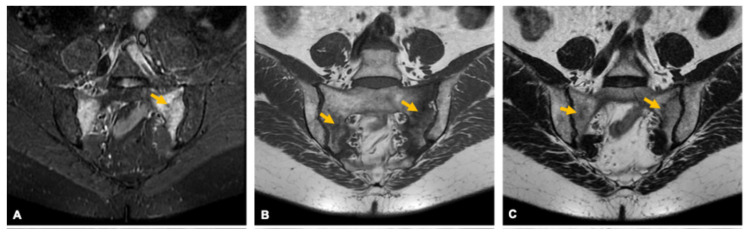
Sacral insufficiency fracture in a 68-year-old woman. Coronal MRI images in STIR (A), T1 (B), and T2 (C) sequences show fracture lines involving both sacral alae, oriented parallel to the sacroiliac joints (arrows), associated with marked bone marrow edema (A).
